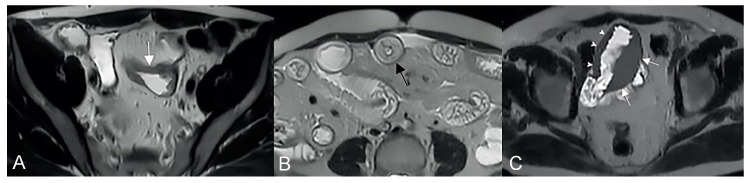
Degree of wall thickening and type of involvement along bowel circumference. (A) Axial T2-weighted image shows mild thickening (<1 cm) of an ileal loop in the pelvis (arrow). (B) Axial T2-weighted image shows moderate symmetric thickening (>1 cm and <2 cm) of a small bowel loop (black arrow), supported by edema of the submucosal layer. (C) Axial T2-weighted image shows marked asymmetric thickening (>2 cm) of an ileal loop in the pelvis (arrows); the other side of the bowel wall is less thick (arrowheads).
